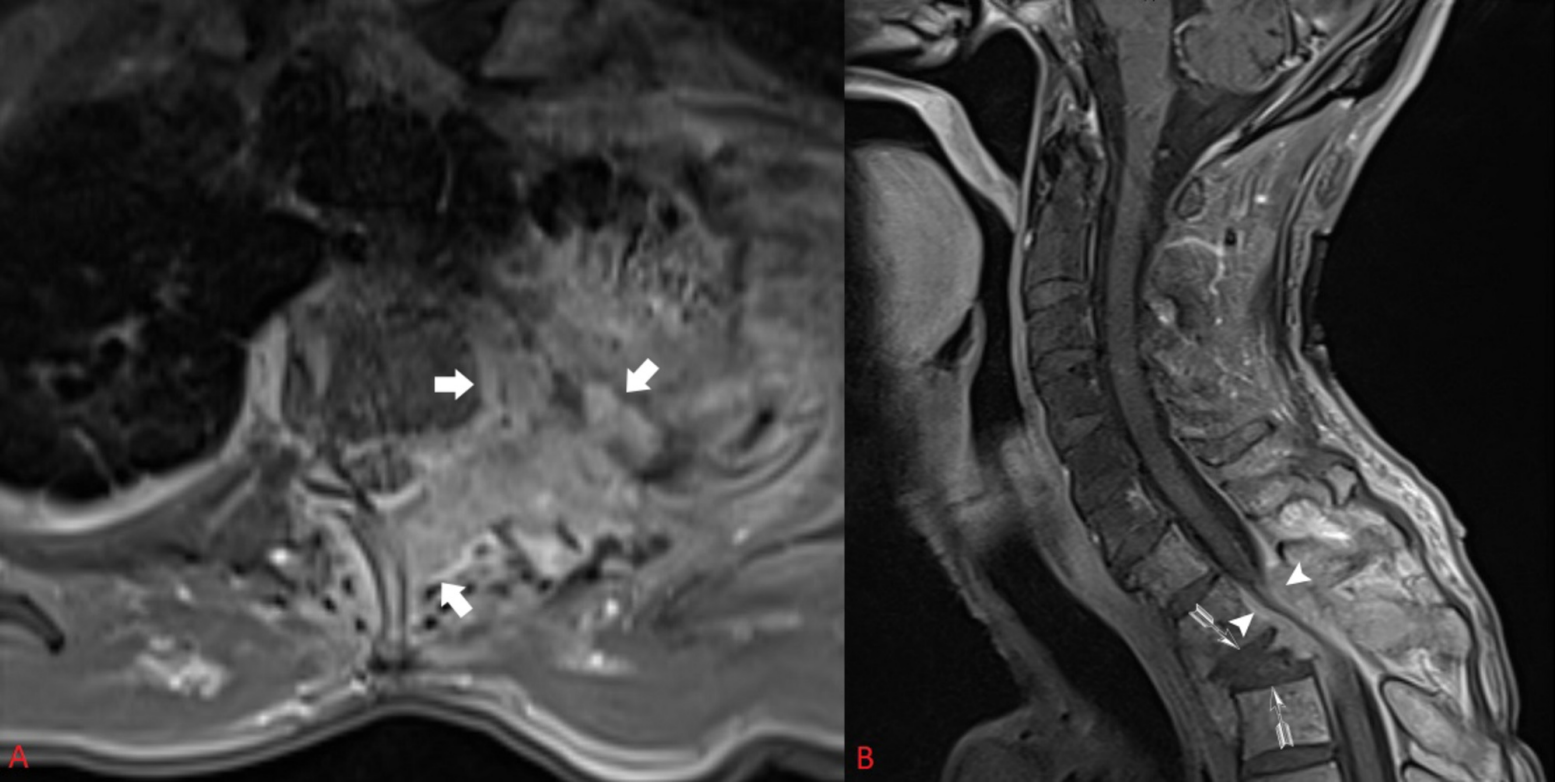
Pre-operative axial (A) and sagittal (B) post contrasted MRI demonstrate a tumor eroding through the chest wall, vertebral body, pedicle, and posterior elements causing compression at the cervical-thoracic junction, kyphotic deformity, and segmental instability Tumor invasion can be seen in the T2 Vertebral Body, Chest Wall, and Posterior Elements on axial imaging (Thick Arrows). Kyphotic deformity is denoted (Feathered Arrows). Spinal Canal Compromise is also shown (Arrow Heads).
